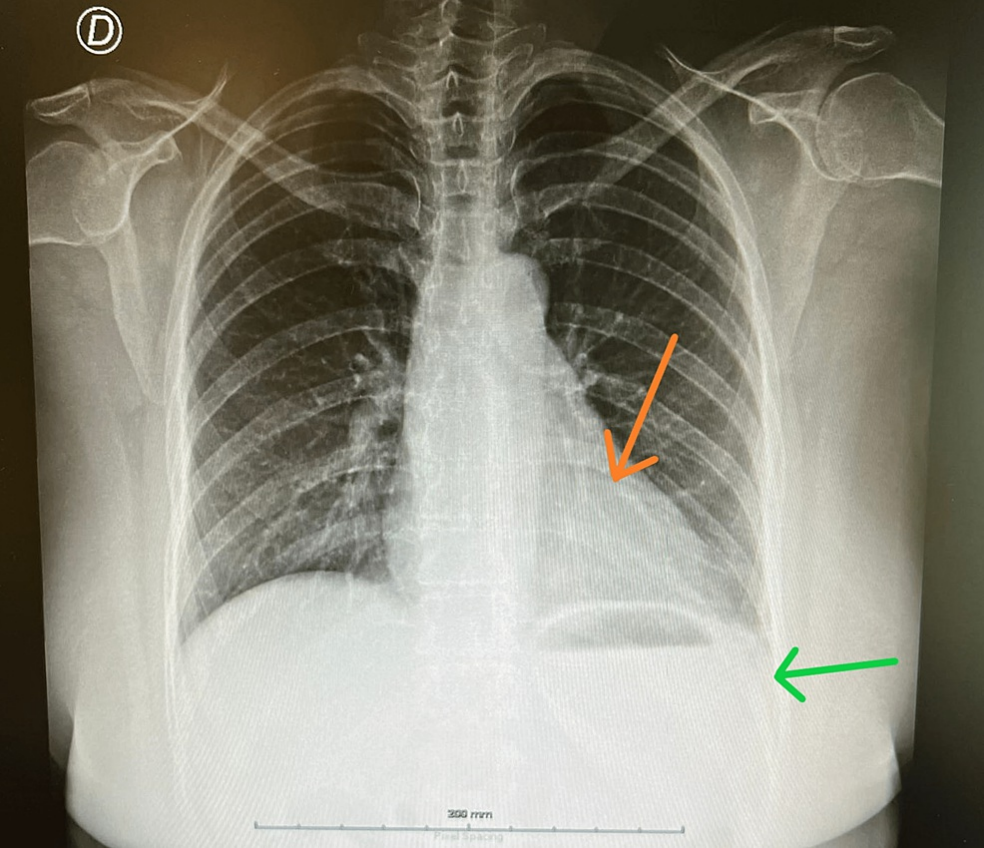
Chest X-ray. Orange arrow indicates a slight increase in cardiothoracic index; Green arrow represents a small pleural effusion on the left thorax.
